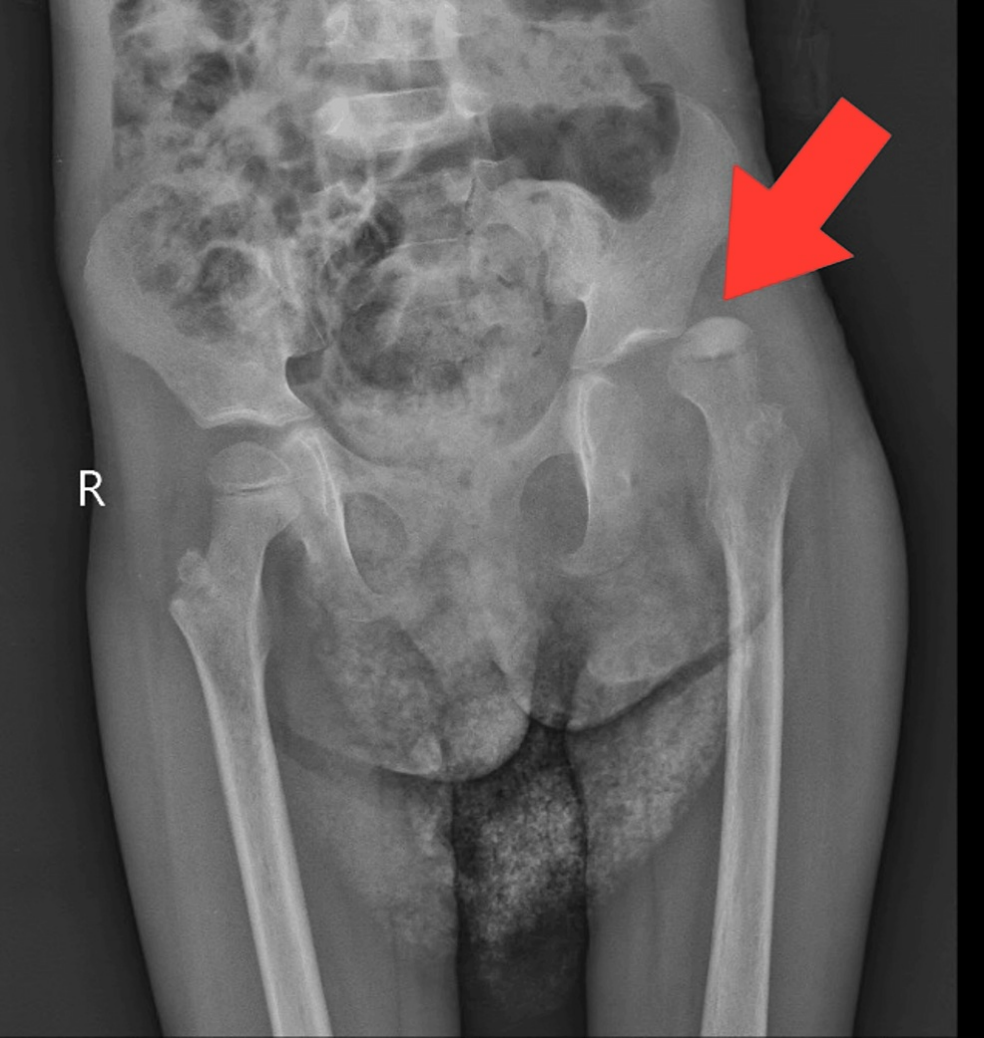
Radiographic imaging of bilateral hip joint
